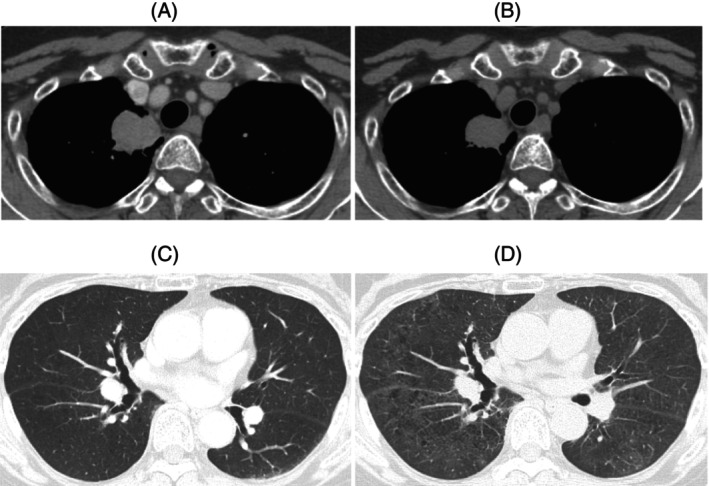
(A) Chest computed tomography before introduction of sotorasib reveals a mass shadow in the right upper lobe. (B) On day 80 after the start of sotorasib, the mass shadow had shrunk slightly. (C) Chest computed tomography before treatment with sotorasib shows no obvious abnormal shadows in the background lungs. (D) On day 80 after the administration of sotorasib, extensive ground glass opacities found in both lung lobes.
